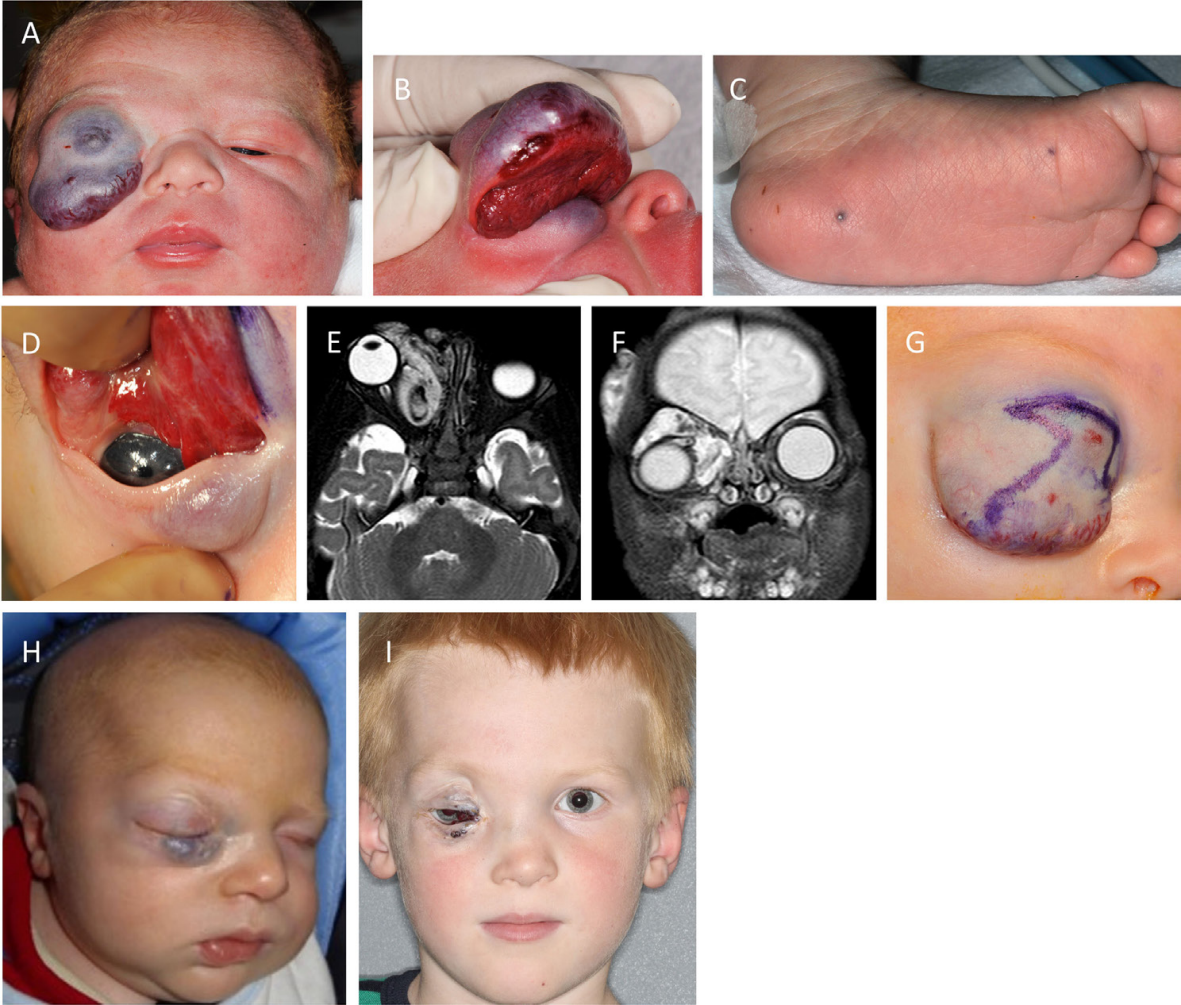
A newborn male with an extensive orbital and periorbital venous malformation (VM) (A,B) and small cutaneous lesions in the feet (C). The lesion massively expanded the upper lid, occluded the visual axis, displaced the globe, and involved the lower lid to a lesser extent (A,B,D). Axial (E) and coronal (F) T2-weighted magnetic resonance imaging showing extensive orbital/periorbital involvement with intracranial communication, and affecting the temporal area. A debulking procedure was performed (G) with significant reduction of the involved upper lid with improvement postoperatively (H). There was gradual expansion of the VM required further debulking procedure 28 months later. The globe remained displaced with minimal vision (I).
